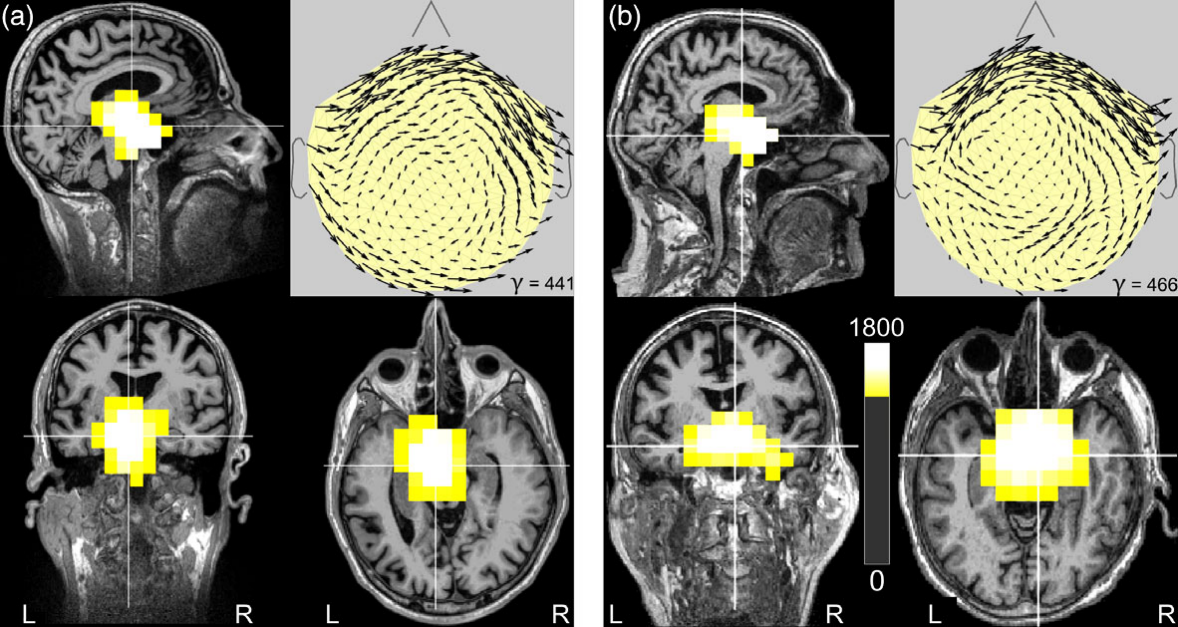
Arrow maps and magnetite locations of the two oldest subjects. Not shown here are the magnetite masses, calculated separately, but which we placed in Figure 8. (a) Upper right: Arrowmap of the particles of the 81 y/o subject. Other three images of a: Inverse solution placed onto the MRI of that subject. (b) The same for the 89 y/o subject. The location of magnetite particles is seen to be similar for these older disease‐free men, as well as their total mass, seen in Figure 8 to be about 12 μg [Color figure can be viewed at http://wileyonlinelibrary.com]
